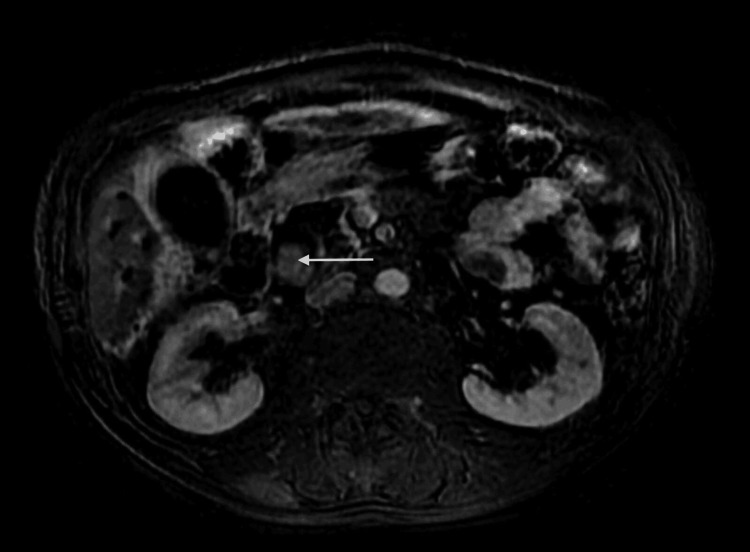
Post-contrast T1-weighted fat-saturated subtraction imaging. Post-contrast T1-weighted fat-saturated subtraction imaging confirms the enhancement of the lesion in the distal common bile duct (arrow).
